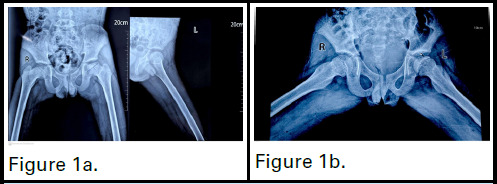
X-ray AP view of the hip showing loss of femoral contour on the acetabulum. Figure 1b. X-ray frog leg view of the Hip showing the femoral head over the acetabulum on abduction.
